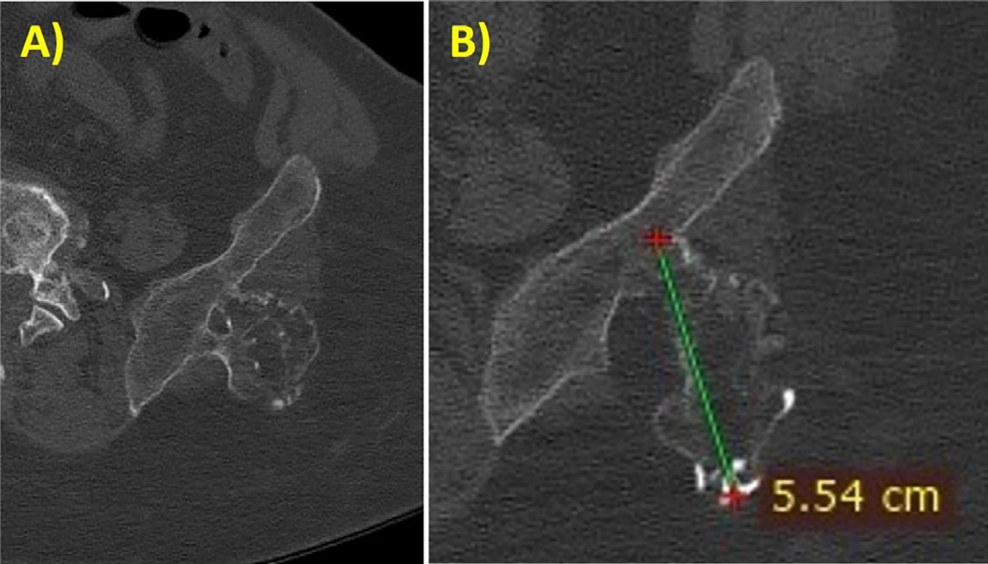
(A, B) CT axial scan showing another bulky growth-bone lesion (maximum diameter of 5 cm) originating from the left ileum.
